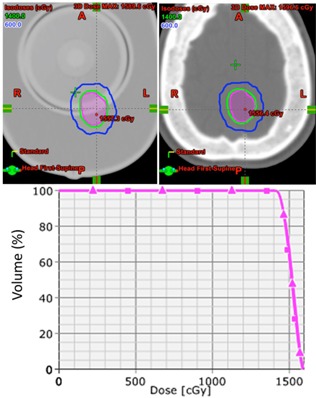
Preplan (top left) generated for a 2 cm target volume (magenta) in 25 min using 10 conformal beams. Treatment plan (top right) generated in less than 5 min using patient CT with the preplan template. DVH (bottom) of the preplan (square) and the template‐generated plan (triangle) demonstrates a good agreement.
